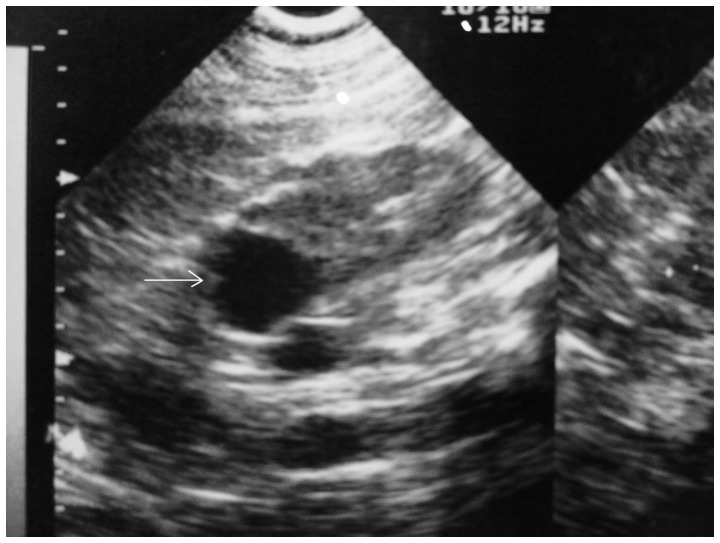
Ultrasonography of the cyst four years previously.
